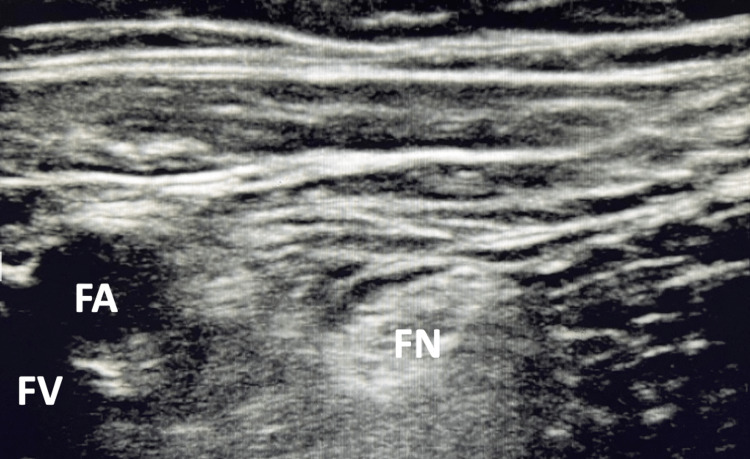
Transverse ultrasound view of the left femoral crease identifying the femoral nerve (FN), artery (FA), and vein (FV)
